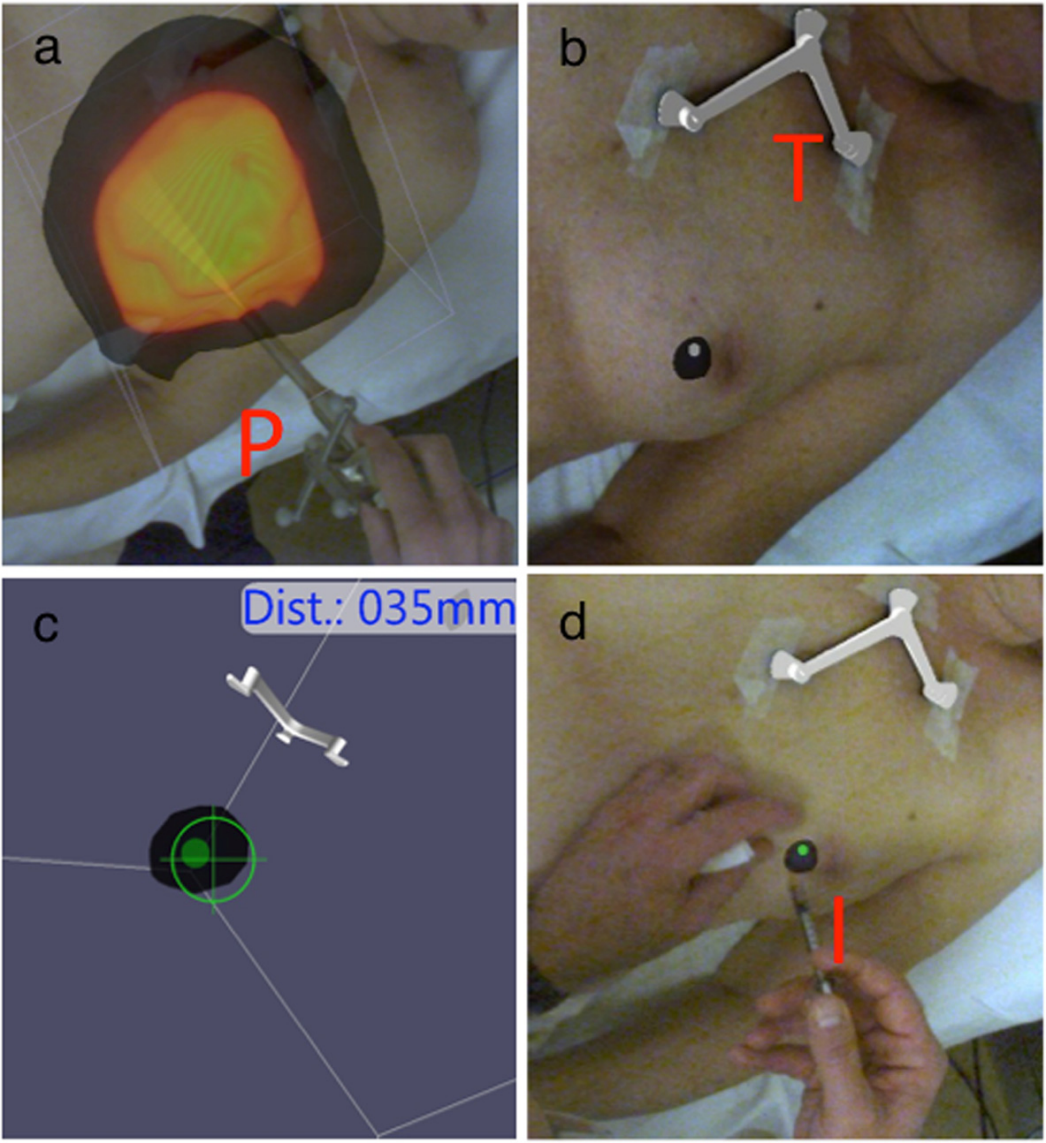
Freehand SPECT method. (a) Data acquisition using the freehand SPECT device; radioactivity is measured with the probe from multiple directions. *P* is the probe, and in yellow, the detection beam of the probe. The orange cloud is an accumulation of the area where it is measured. (b) The localisation of the ^125^I seed after reconstruction. The ^125^I seed reconstruction is projected over the optical image in purple. *T* is the patient tracker. (c) 3D visualisation of the distance and direction of the probe tip to the ^125^I seed. (d) Injection of ^99m^Tc-nanocolloid guided by freehand SPECT. *I* is the tracer injection localisation.
